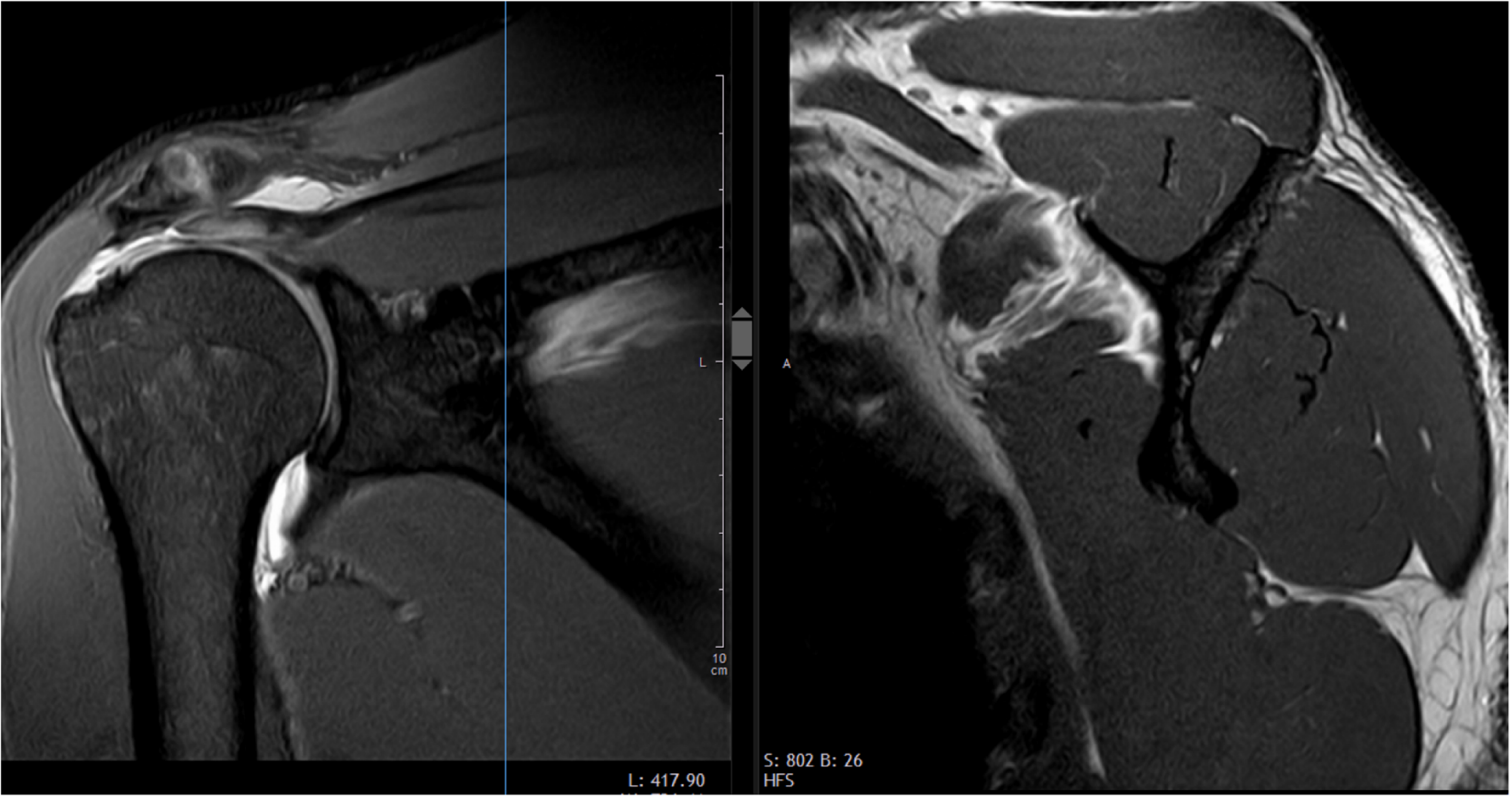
Coronal and sagittal MR images of the right shoulder of a 37-year-old man showing a full-thickness supraspinatus tendon tear. The examination was made seven weeks after a fall on the shoulder following a header duel during a football match. Despite the young age, immediate pain, initial pseudoparesis, and normal muscle trophics, the insurance expert claimed that the accident had only caused a contusion and that the tendon was already torn before the event
